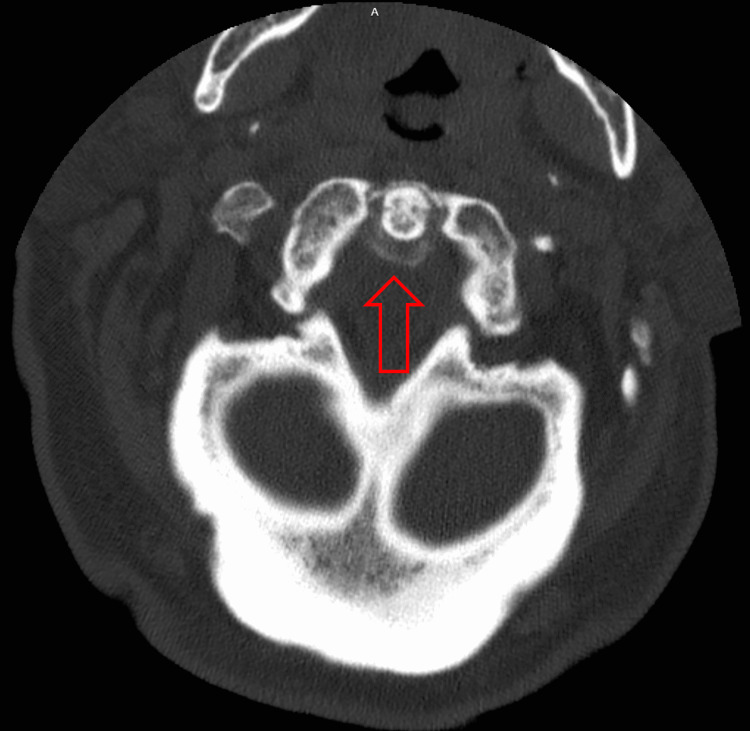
CT neck axial view demonstrating area of calcification around the odontoid process (red arrow).
